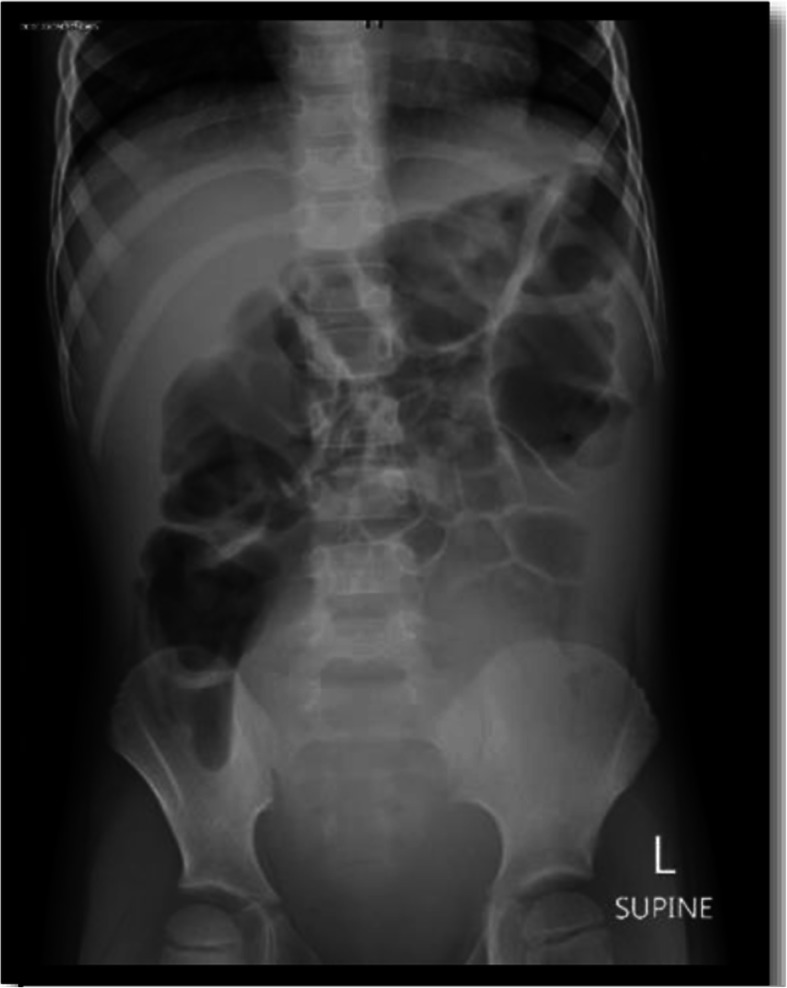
Abdominal Xray showing gas distension of the transverse colon
